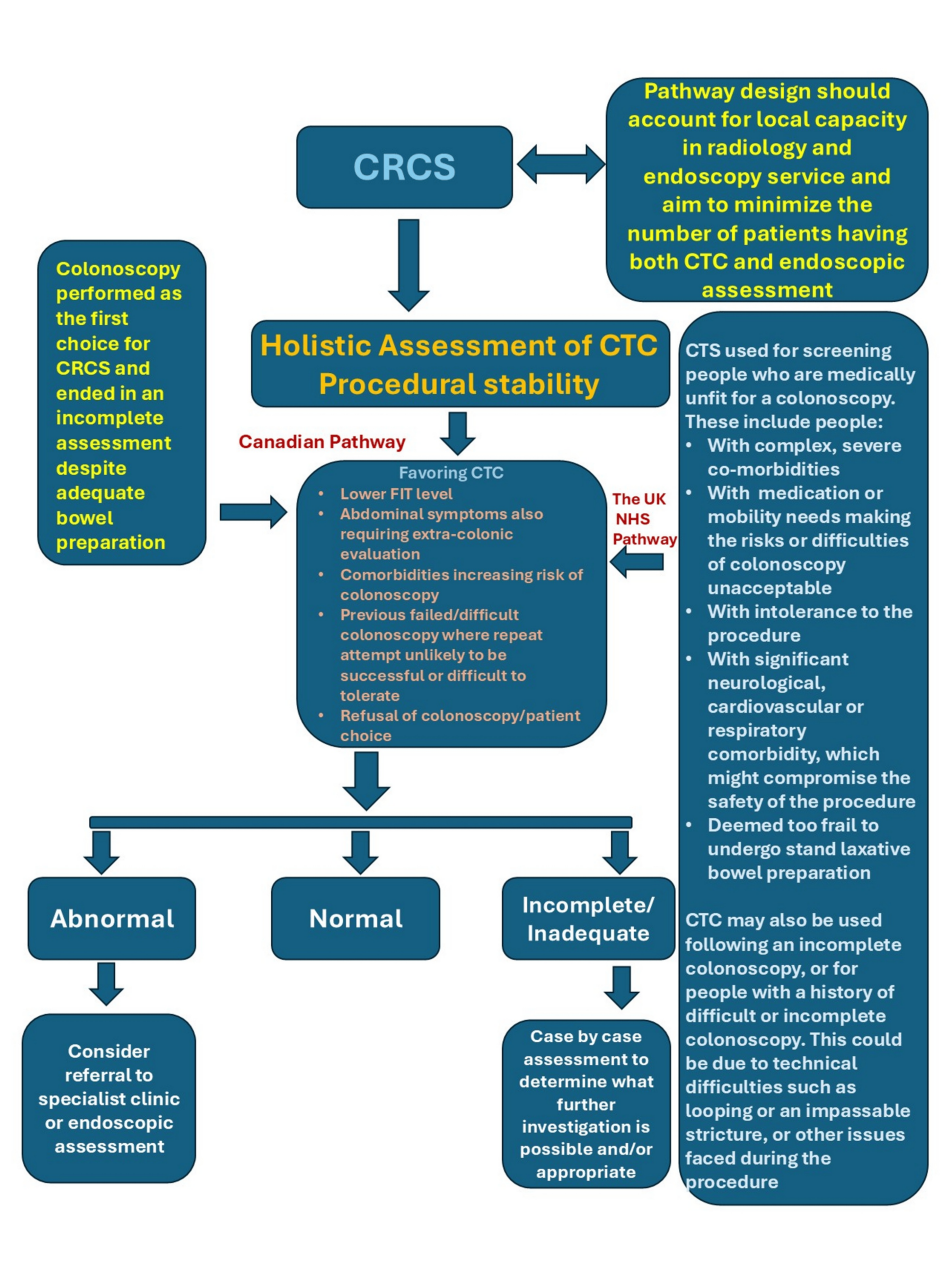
Appropriate Use of CT Colonography (CTC) in Colorectal Cancer Screening (CRCS) Based on Canadian and the United Kingdom National Health Care Practice Guidelines Synthesized by authors from the discussion above from [69,70]. "Since these concepts were developed in two countries with fewer resources that the USA with national health systems conscious of the need for efficiency and cost-benefit' considerations, these ideas can be useful in resource-constrained states like Mississippi"
